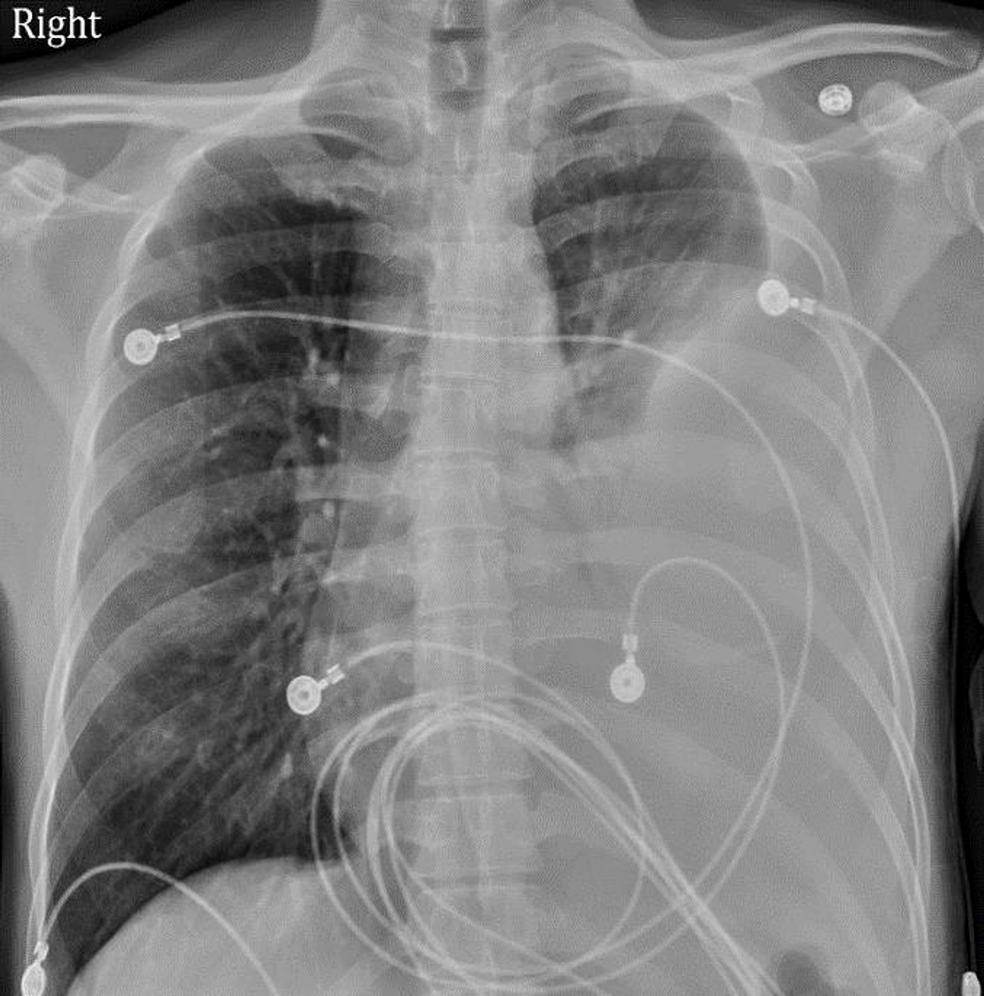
Chest X-ray showing large left pleural effusion with associated airspace disease.
